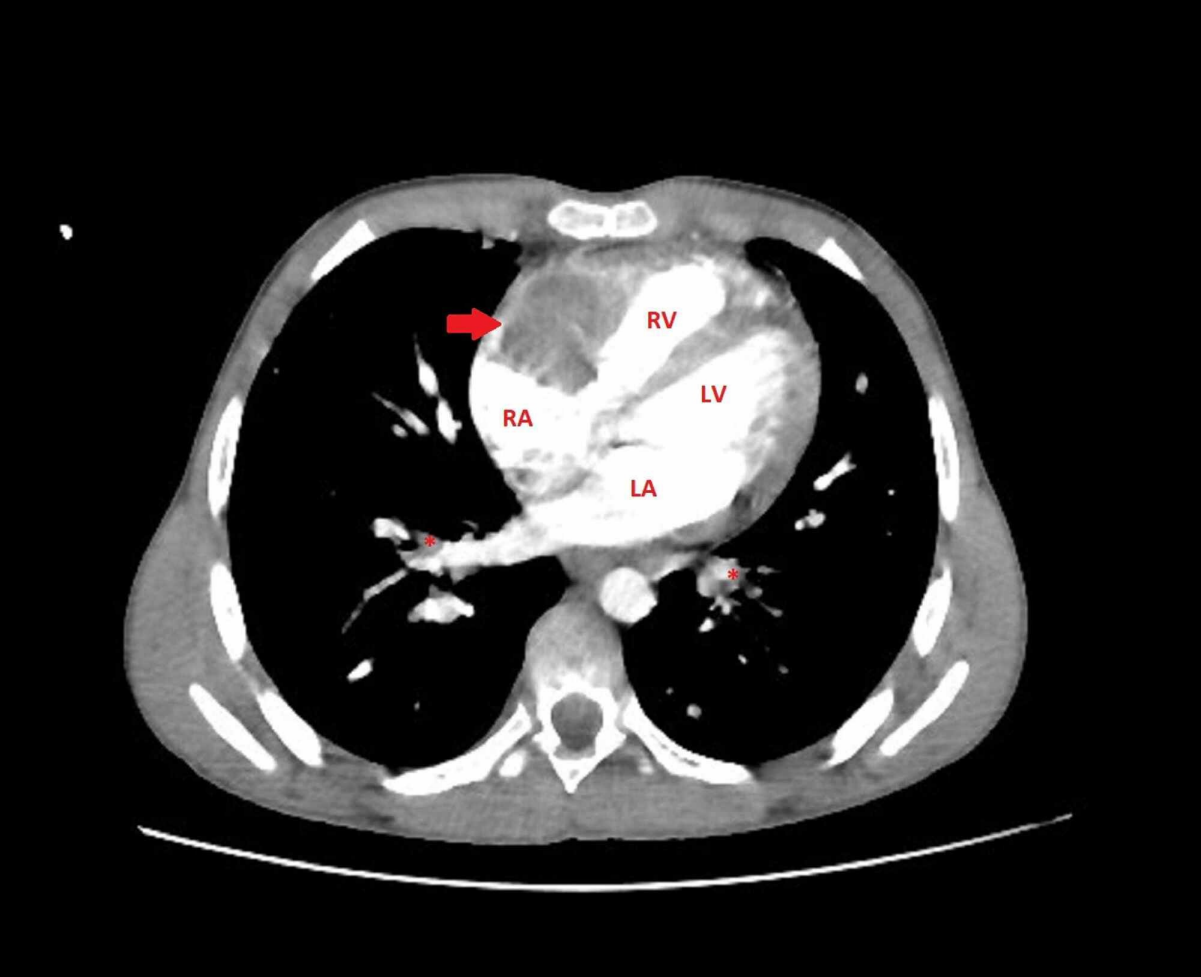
Chest CT scan with contrast showing a well-defined hypodense mass (red arrow) of 35 mm x 32 mm, partially calcified that straddles between the RA and the RV, associated with similar pulmonary lesions located in the segmentary branches of the left inferior lobar artery marked with a red asterisk. RV, right ventricle; RA, right atrium; LV, left ventricle
